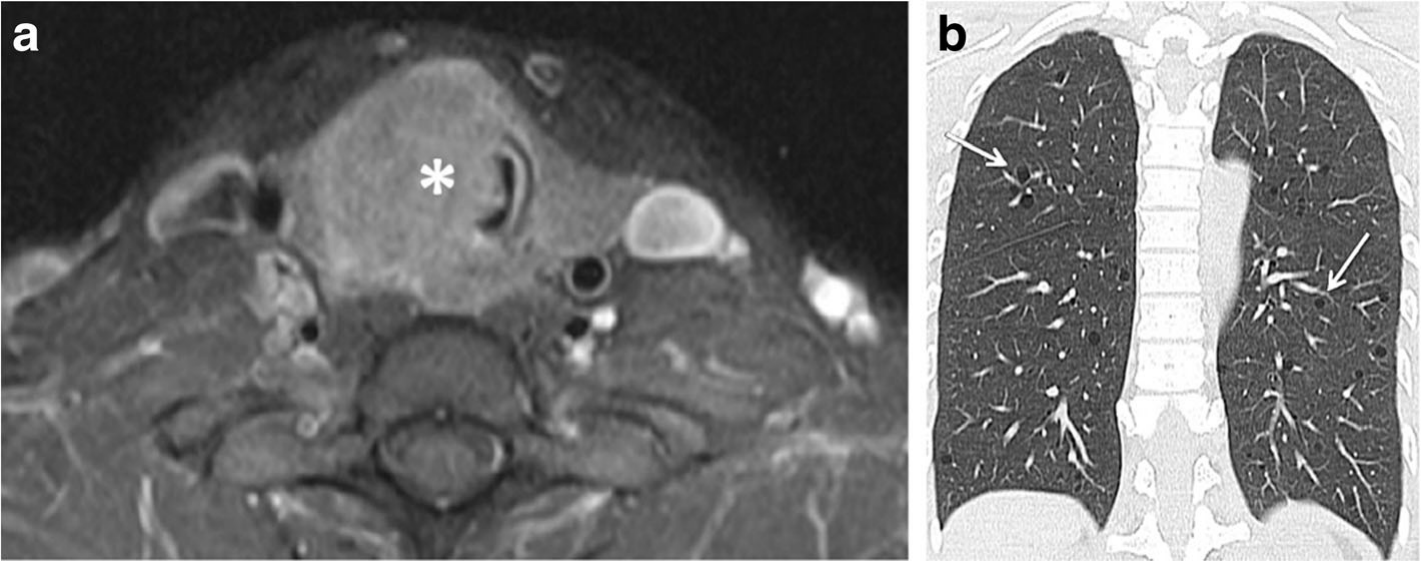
Neck CT scan (axial reconstruction) showing a large right sided thyroid mass (asterisk) which caused marked tracheal deviation and stenosis (a). Thorax CT scan (coronal reconstruction) showing multiple cystic lesions with thin wall in both lungs (arrows) (b)
